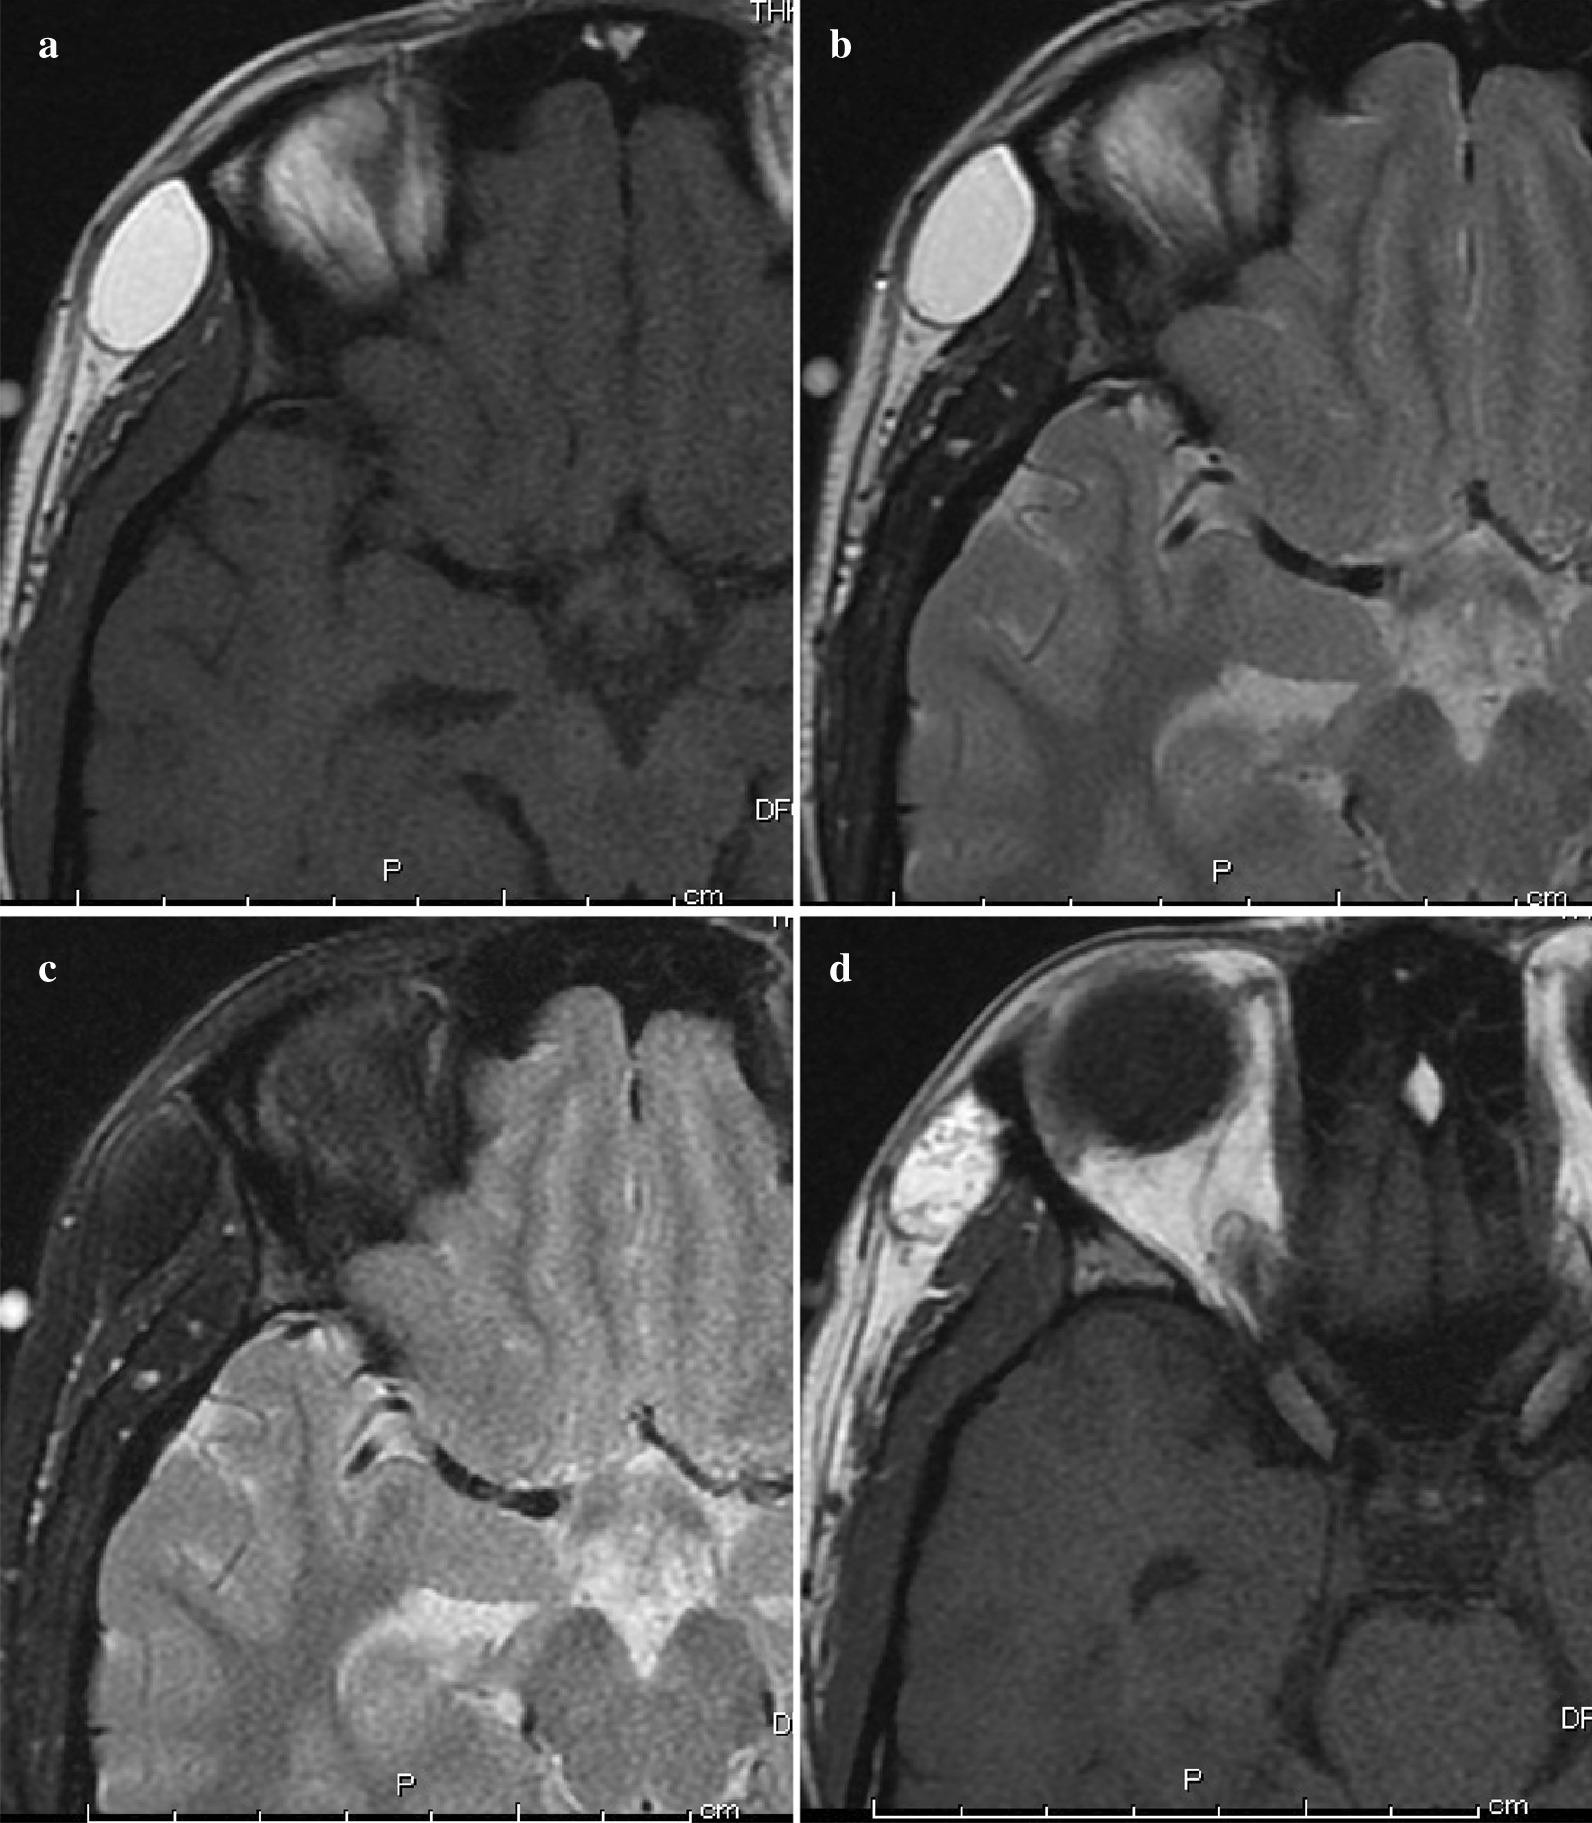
Magnetic resonance imaging before surgery. a T1-weighted image of the lesion. The tumor shows homogenous high intensity compared to the brain. A thin capsule is observed around the tumor. b T2-weighted image of the lesion. The tumor shows homogenous high intensity compared to the brain. This image also indicates a thin capsule. c Fat-suppressed T2-weighted image of the lesion. The internal signal from the tumor is almost completely suppressed compared to the normal T2-weighted image. d Another slice of the T1-weighted image of the tumor. The internal signal of the tumor is not homogenous when compared with the slice presented in a. This finding is thought to be atypical of a lipoma
